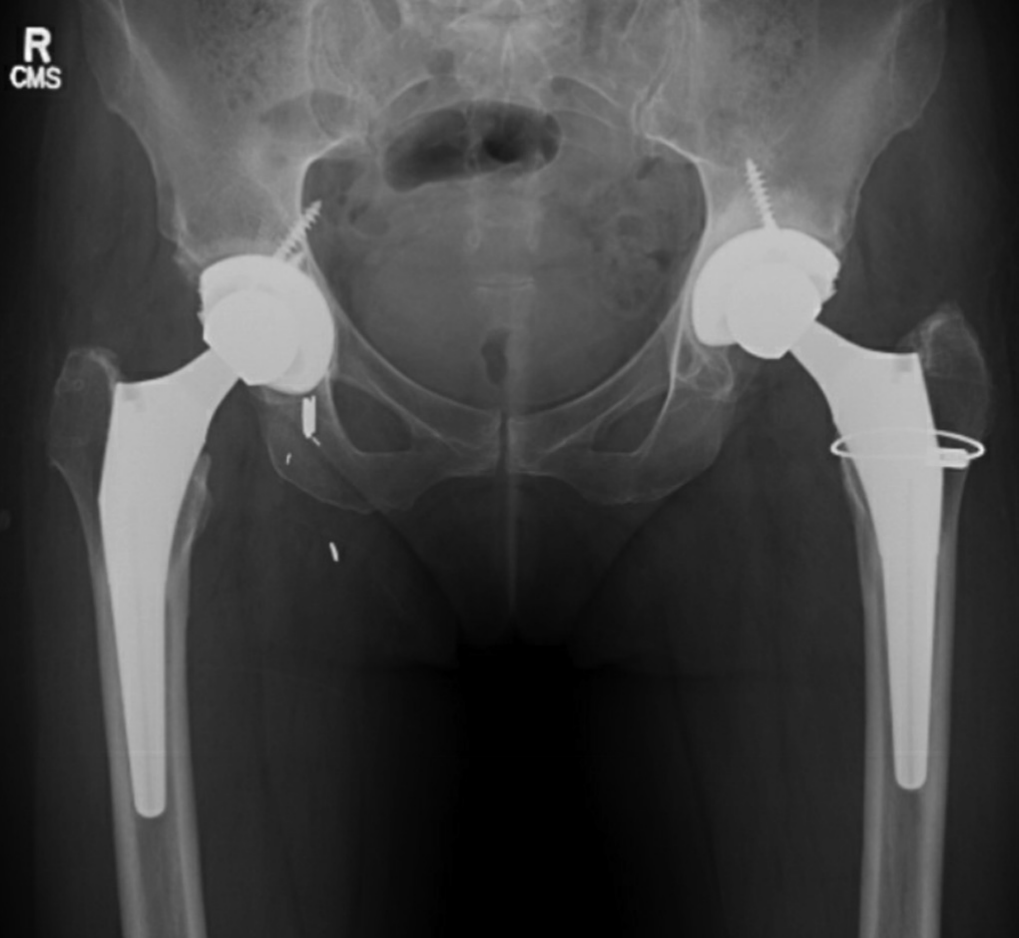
Postoperative pelvic radiograph 7 years after first-stage L-THA, March 2017.
